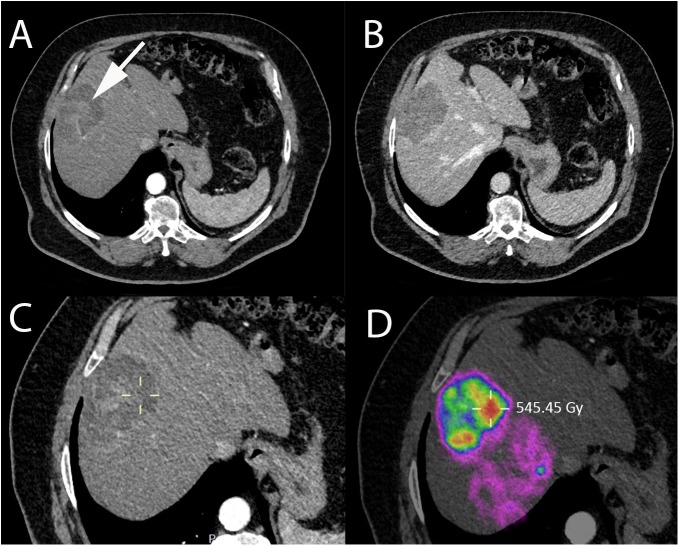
Immediate post-SIRT dosimetry using quantitative PET-CT with internal pair positron emission by ^90^Y in a 73-year-old patient with non-alcoholic steatohepatitis and HCC. (A) Contrast-enhanced CT-scan acquired during arterial phase showing HCC in segment VIII with heterogeneous areas of enhancement (white arrow). (B) Contrast-enhanced CT-scan acquired during portal phase showing contrast wash-out of the lesion. (C) Contrast-enhanced CT (arterial phase) and (D) quantitative PET-CT performed after SIRT with Y^90^-microspheres allowing direct assessment of the administered dose, expressed in Gray (Gy) using a region of interest (yellow cross).
